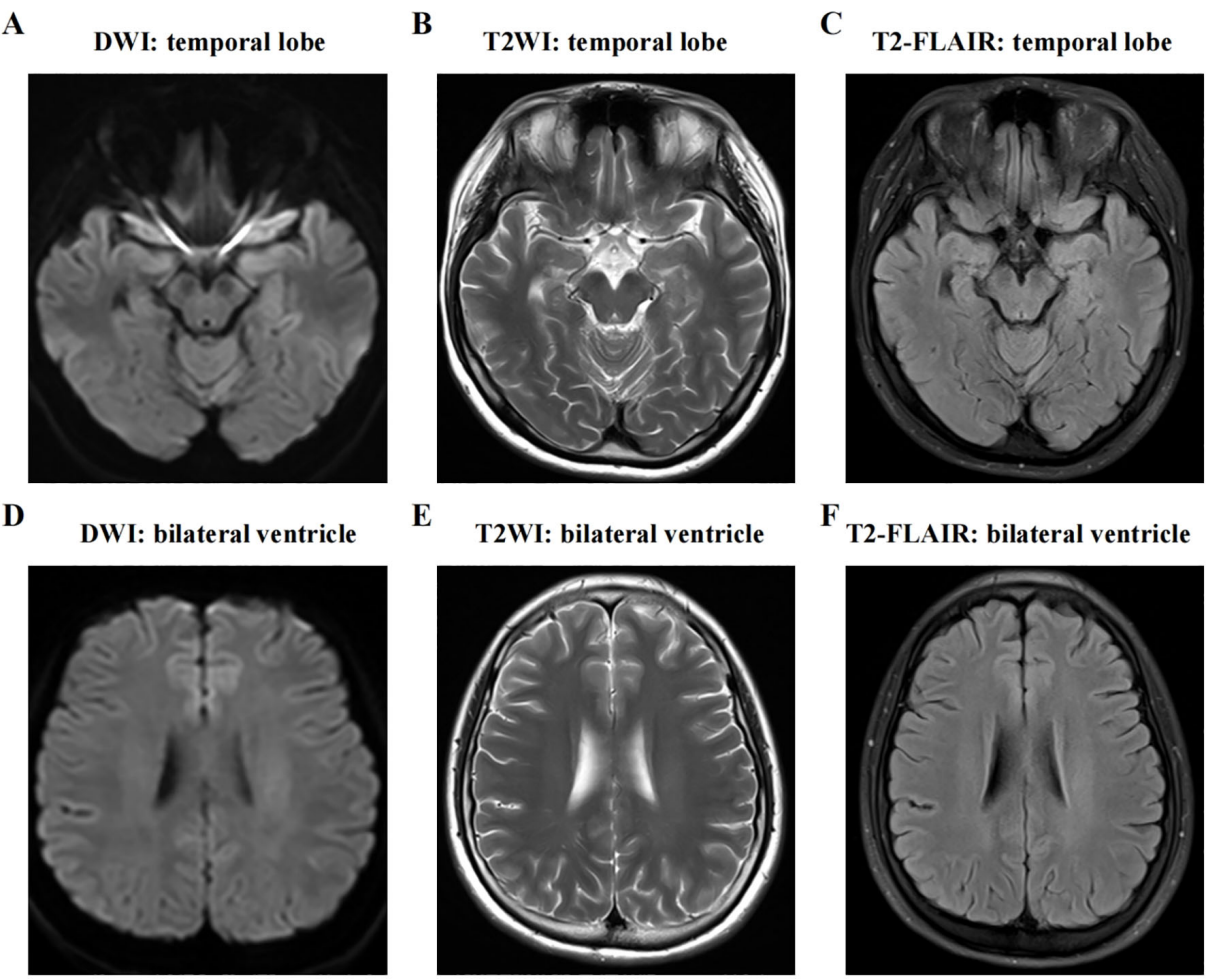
MRI of the brain shows no abnormal signs. The two hemispheres of the brain are symmetrical, with clear distinction between gray matter and white matter. No obvious abnormal signal is detected on axial T1WI, T2WI, FLAIR, or DWI sequences. (A-C) T1WI, T2WI, and T2-FLAIR axial view presenting medial temporal lobe; (D-F) T1WI, T2WI, and T2-FLAIR axial view presenting lateral ventricle. DWI, diffusion weighted imaging; T2WI, T2-weighted imaging; T2-FLAIR, T2-weighted fluid attenuated inversion recovery.
